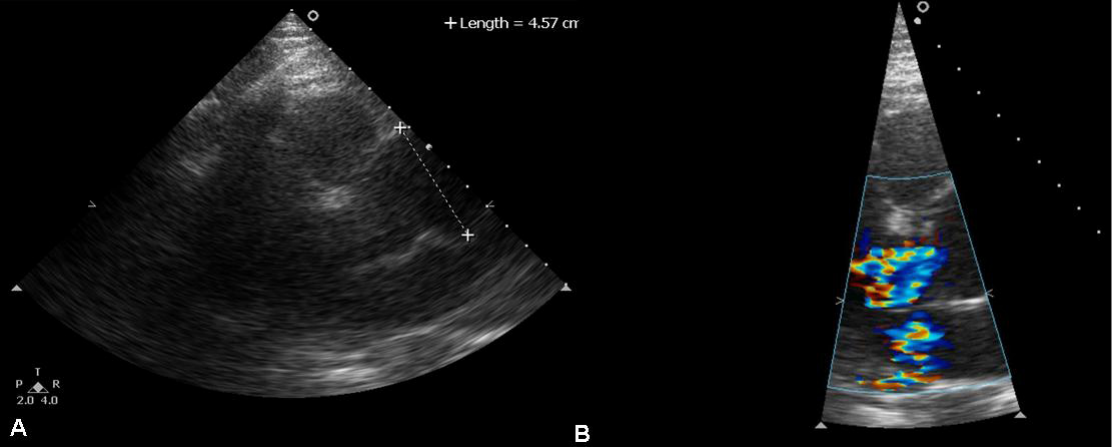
Transthoracic echocardiography (TTE): Panel A depicts long axis parasternal view with a dilated aortic root of 4.57 cm, without pericardial effusion. Panel B the use of Color Flow Doppler unveiled a moderate aortic and mild mitral regurgitation.
